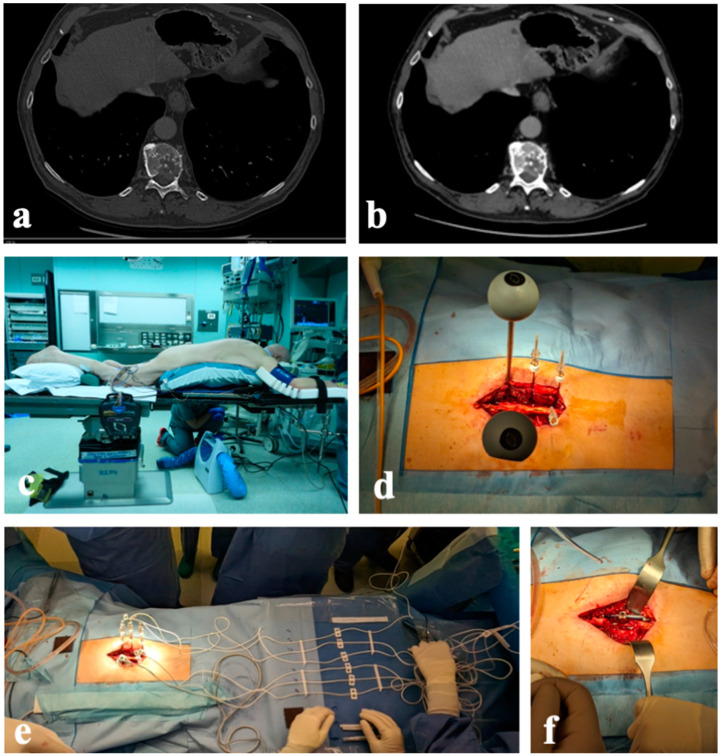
Case 3. A 73-year-old man with T11 metastasis from leiomyosarcoma grade 3: (a,b) axial CT scan shows the lytic lesion with spinal cord compression; (c) positioning of the patient; (d) insertion of the electrodes; (e) connection of the electrodes with the pulse generator; (f) definitive instrumentation and laminectomy.
